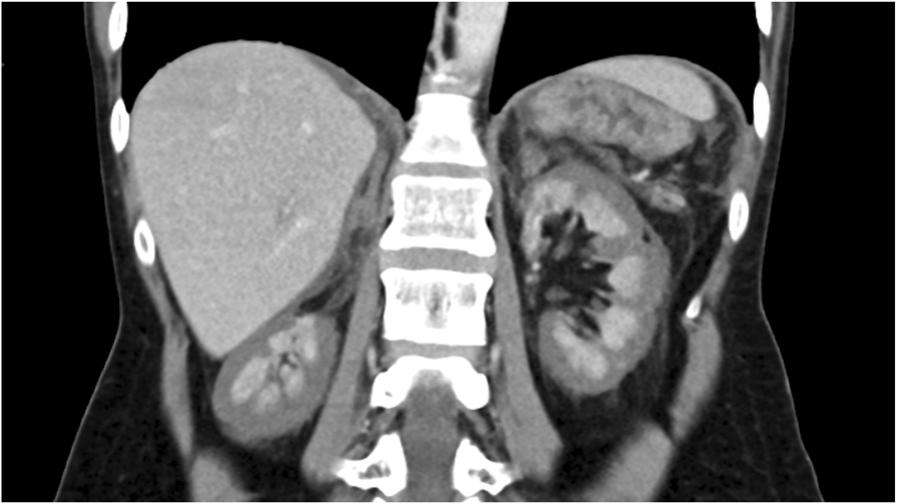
Contrast-enhanced abdominal CT scan, with a bilateral hypo-perfused renal cortex and an adequately perfused renal medulla = “reverse rim sign”. This finding is pathognomonic for acute renal cortical necrosis
